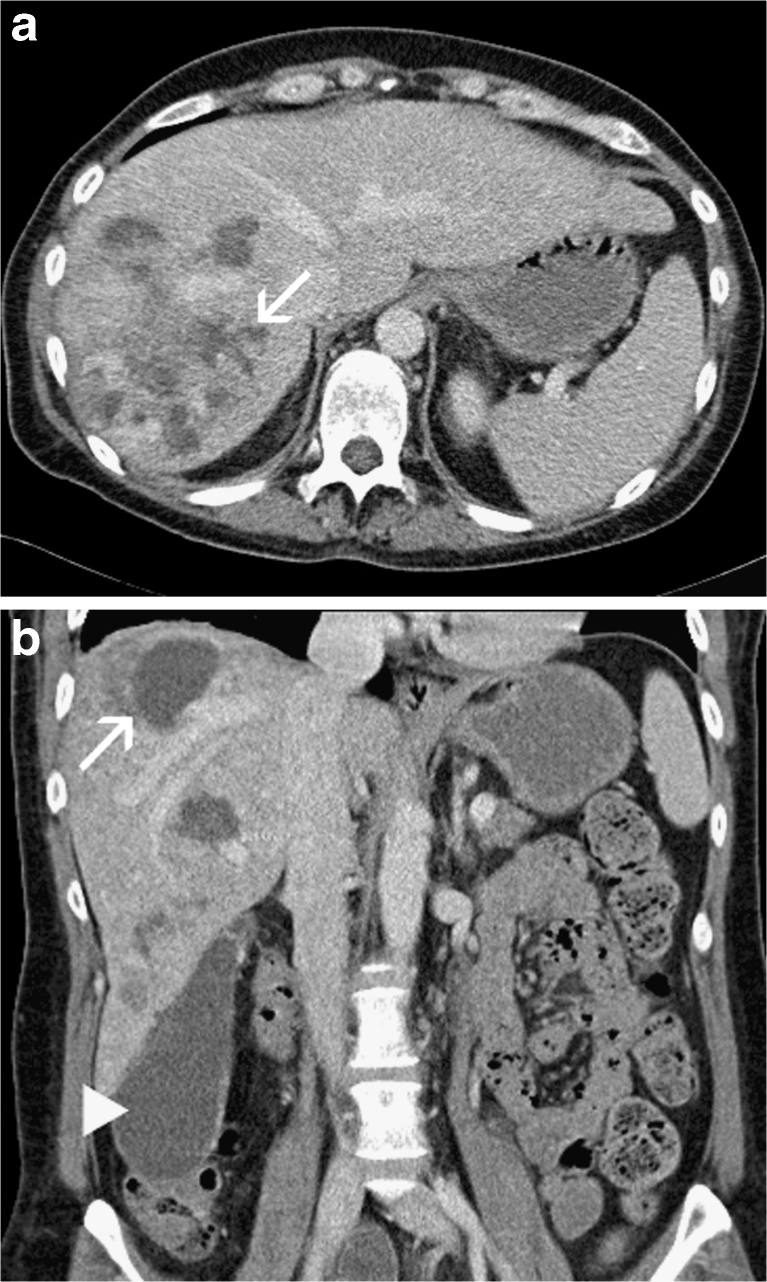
CT in the portal venous phase, axial section (a) and coronary reconstruction (b) showing multiple biliary abscesses (*arrows*) and hydrops of the gall bladder (*arrowhead*) 22 weeks following hepatic artery embolisation (HAE)
